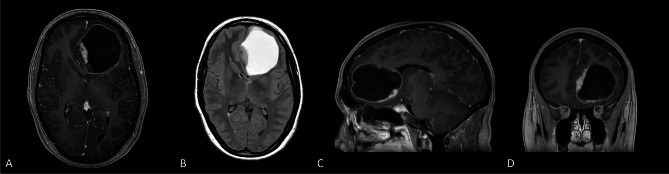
Axial T1 post-contrast (A), axial T2 FLAIR (B), and sagittal (C) and coronal (D) T1 post-contrast MRI brain demonstrating a large, well-circumscribed cystic left frontal mass with an enhancing nodule situated on the inferomedial aspect in a 25 year old woman with headaches and blurry vision. Despite local midline shift and considerable mass effect, there is little perilesional edema. On FLAIR (B), the cyst contents are hyperintense to CSF, likely reflecting higher protein content
